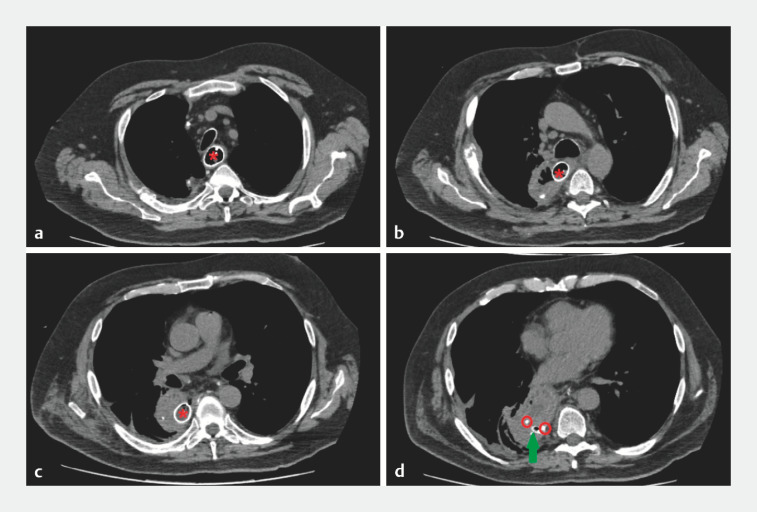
Computed tomography images from the initial scan showing:
